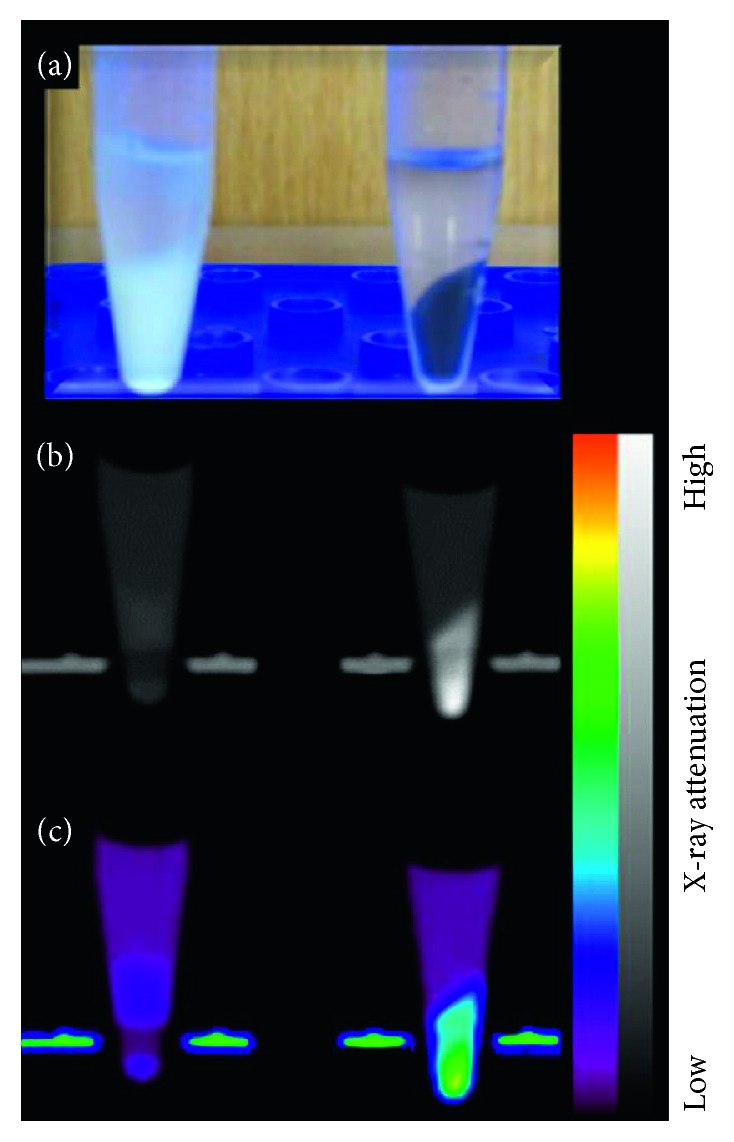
(a) Photograph of the unlabeled MSCs (left) and Bi_4_C@US-tube-labeled MSCs (right). (b) Conventional black and white CT images (coronal view) and (c) color CT images.
